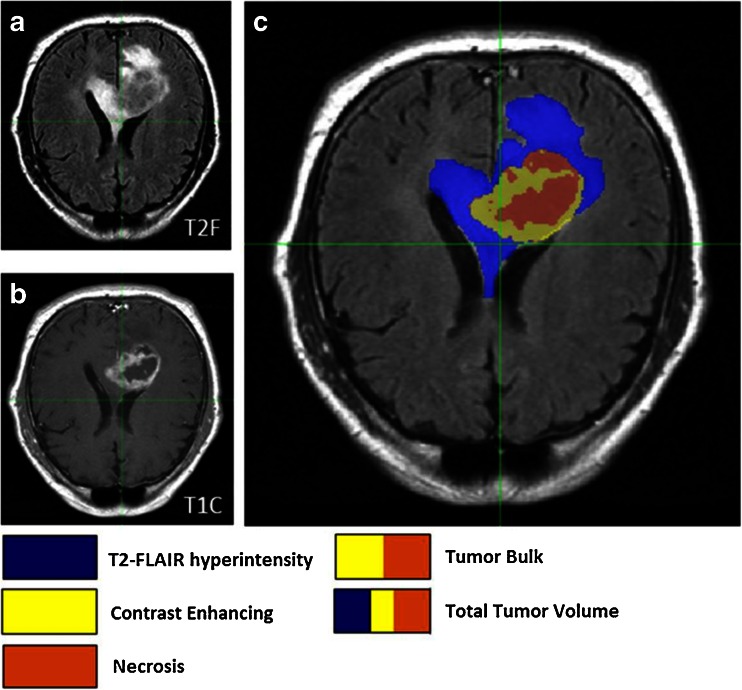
Visualization of naming conventions for the tumor volumetric features used throughout this article for TCGA-02-0033, a 54-year-old male glioblastoma patient. a Tumor-associated T2-FLAIR hyperintensity and total tumor volume was quantified from the T2-weighted FLAIR images. b Necrosis, contrast enhancing, and tumor bulk volume was quantified from T1-weighted post-Gd contrast (T1C) images. c This panel displays both images co-registered and overlayed on top of each other for visualization purposes. Tumor bulk is defined as the total abnormal tumor area on the T1C images: combination of contrast enhancing and necrosis volumes. Total tumor volume is defined as the combination of the tumor bulk and T2-FLAIR hyperintensity volumes
